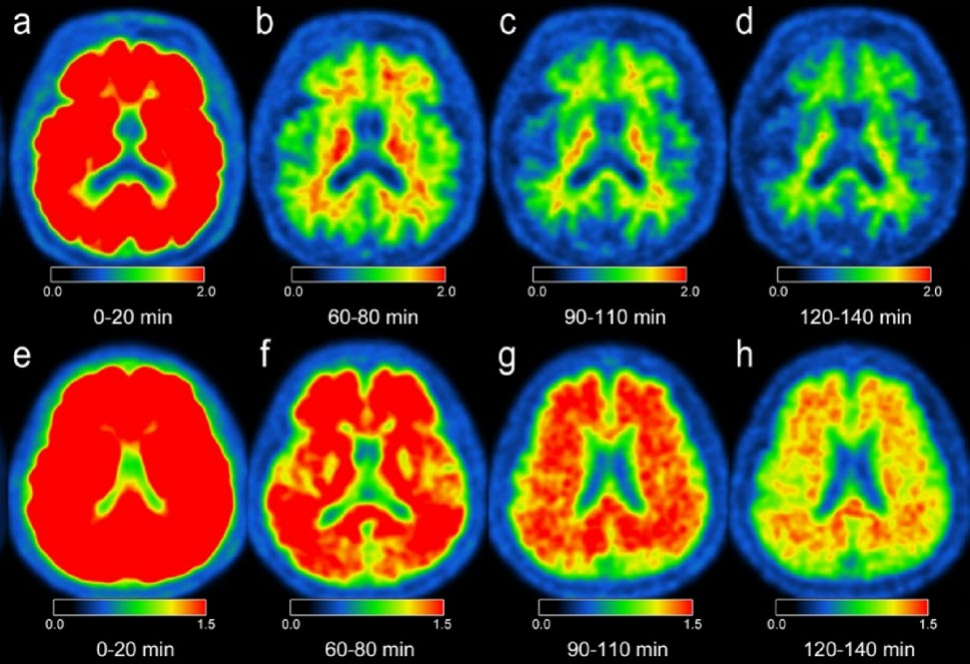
Dynamic amyloid PET/MRI. Dynamic amyloid PET/MRI was performed to minimize the impact of differences between imaging modalities. Representative cases of amyloid-negative (an 85-year-old man; a–d) and amyloid-positive (a 69-year-old woman; e–h) subjects are shown. Images were acquired at 0 min (a, e), 60 min (b, f), 90 min (c, g), and 120 min (d, h) post-injection. Tracer accumulation persists longer in the amyloid-positive case (h) than in the amyloid-negative case (d)
